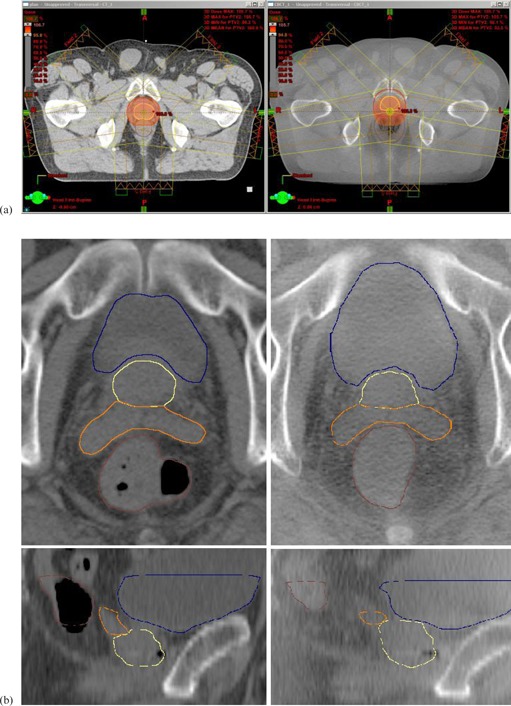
Comparison (a) of planning CT scan (left) and CBCT scan (right) with prostate (CTV2 – yellow line, PTV2 – red line, 1 cm margin) and rectum (brown line) delineated; isocentric transversal slice. Color wash is set to 95% of the dose prescribed to prostate. Using BL‐based alignment, excessive rectal volume causes the prostate movement anteriorly, which leads in prostate underdosage. Lower image quality (b) of CBCT (right) comparing to planning CT (left). Prostate (yellow line), seminal vesicles (orange line), rectum (brown line), and bladder (blue line) are shown in transversal view (upper part) and sagittal view (lower part).
